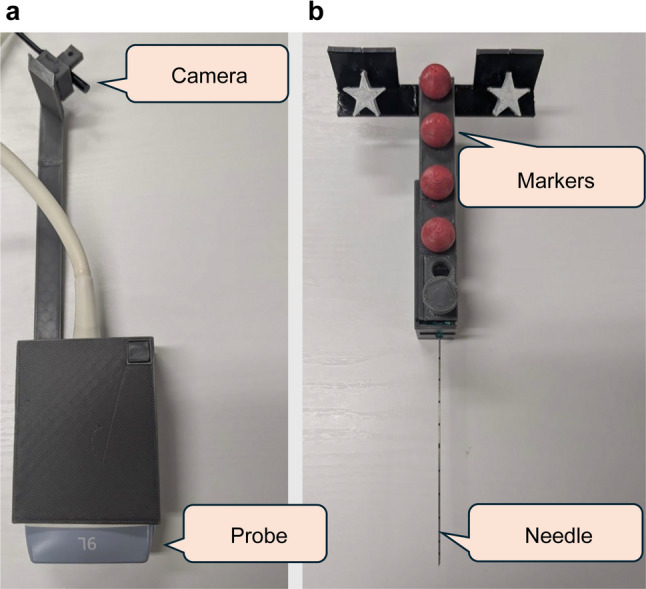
System components. Camera attached to ultrasound probe (a). (b) Puncture needle with six markers: two star-shaped and four circular for real-time positional detection
